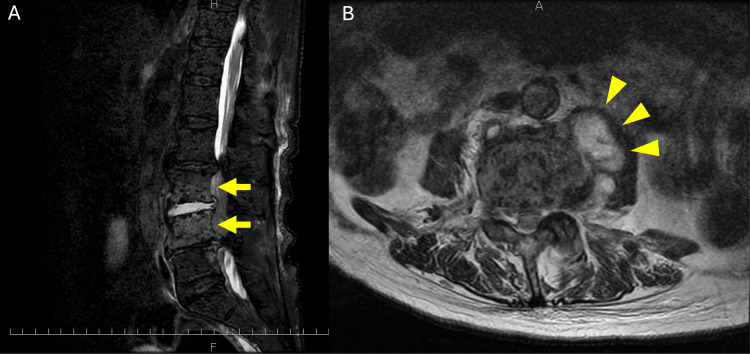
Lumbar magnetic resonance imaging scan. (A) T2-weighted iterative decomposition of water and fat with echo asymmetry and least-squares estimation imaging showing high signal at L3/4 (arrows). (B) T2-weighted fast recovery fast spin-echo revealing imaging paravertebral mass (arrows).
